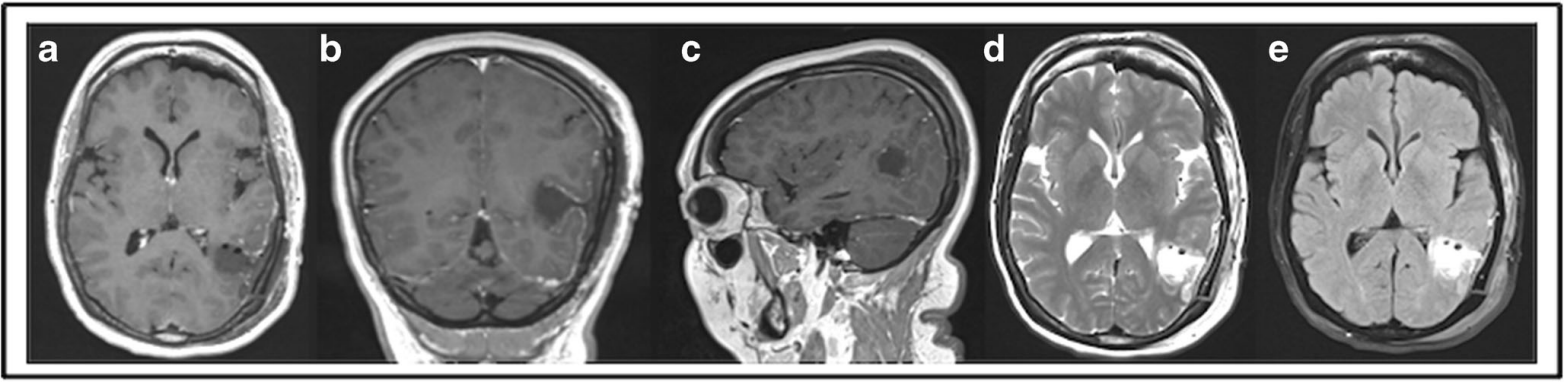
Postoperative MRI. Complete resection (gross total resection). a Axial T1 GAD. b Coronal T1 GAD. c Sagittal T1 GAD. d Axial T2. e Axial FLAIR
